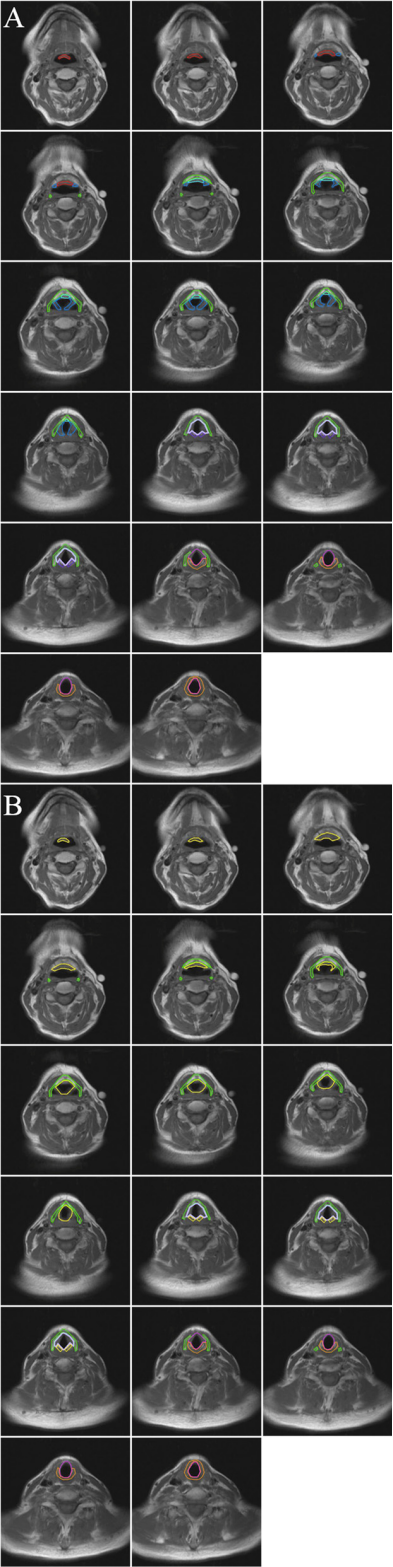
Atlas of the larynx and its substructures on consecutive axial magnetic resonance imaging (MRI) slices, T1-weighted, pre-contrast sequence: the thyroid cartilage is depicted in green, the cricoid cartilage in orange, the arytenoid cartilages in purple, the suprahyoid epiglottis in red, the infrahyoid epiglottis in cyan, the aryepiglottic fold/false vocal folds in blue, the supraglottic larynx in yellow, the glottic larynx in lavender, and the subglottic larynx in magenta. (A) Individual substructures of the larynx. (B) Major divisions of the larynx.
